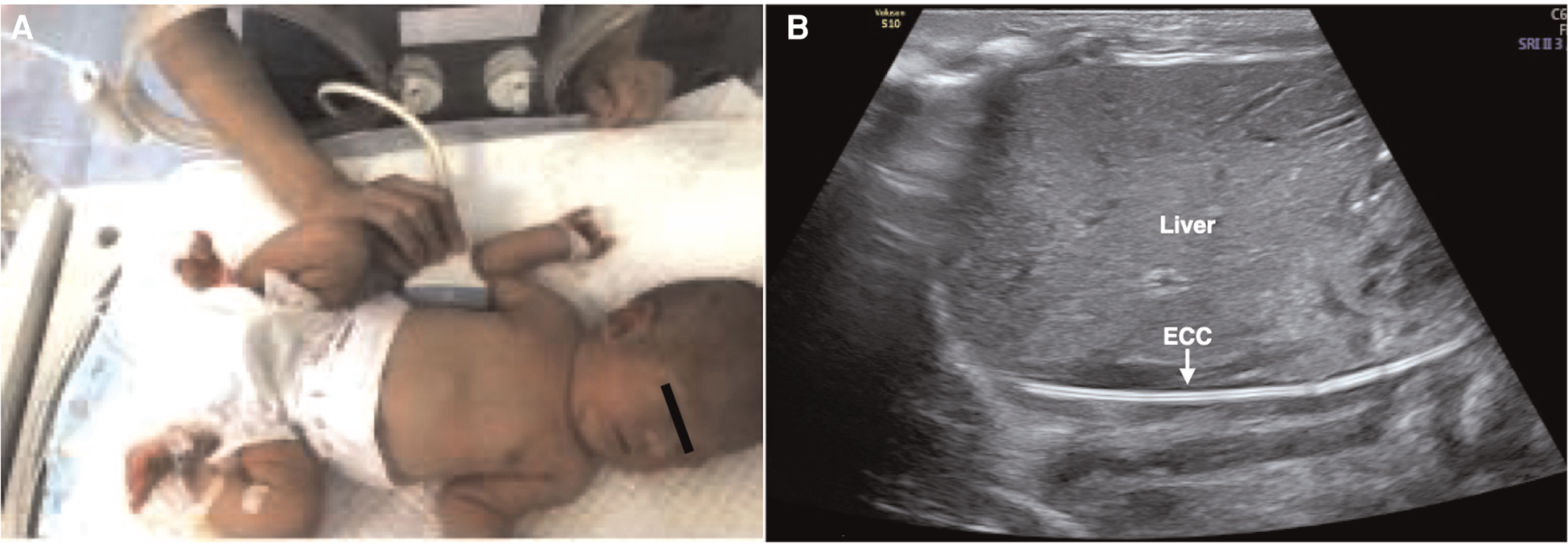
To determine whether the ECC catheter is located in the inferior vena cava. Right midaxillary line view. The probe was placed in the midaxillary line. The baby was placed in the supine position (A), and the ECC catheter showed a high echo “equal-like sign” (“=” line) appearance under ultrasound (B). There were no abnormal conditions, such as folding back of the catheterization (B).
